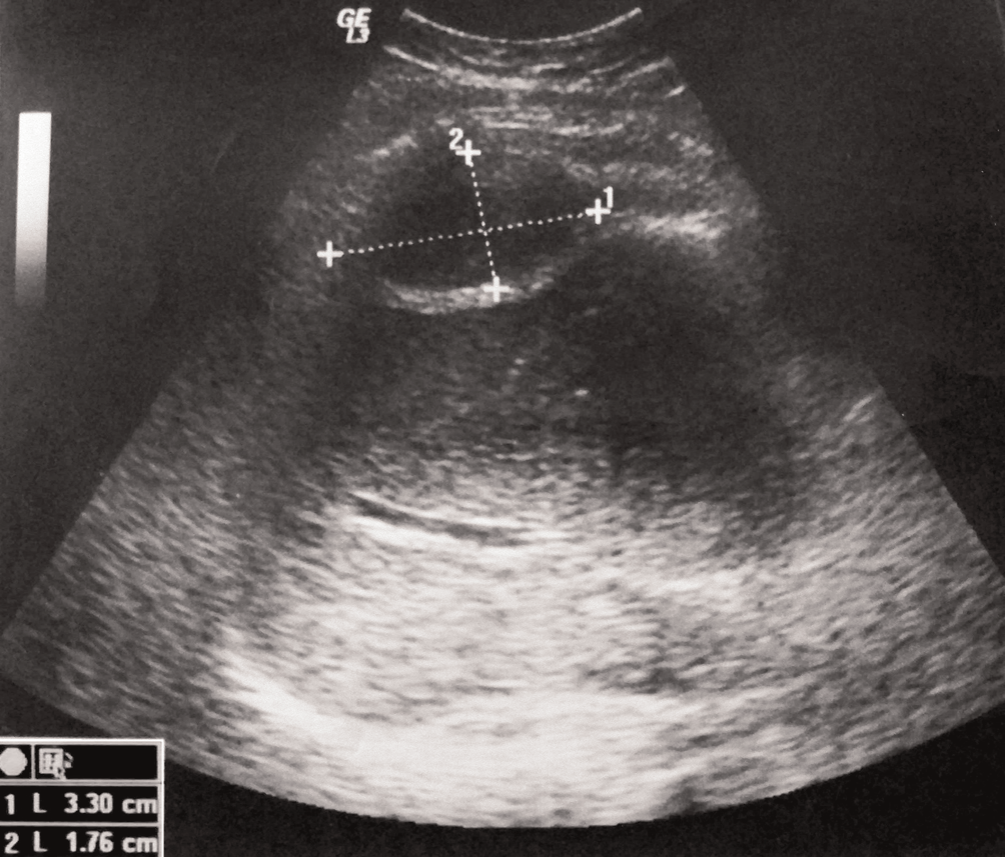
Ultra sonography showed nearly a total vanishing of the biloma, with persistence of a little collection measuring (3.30 × 1.76 cm).
